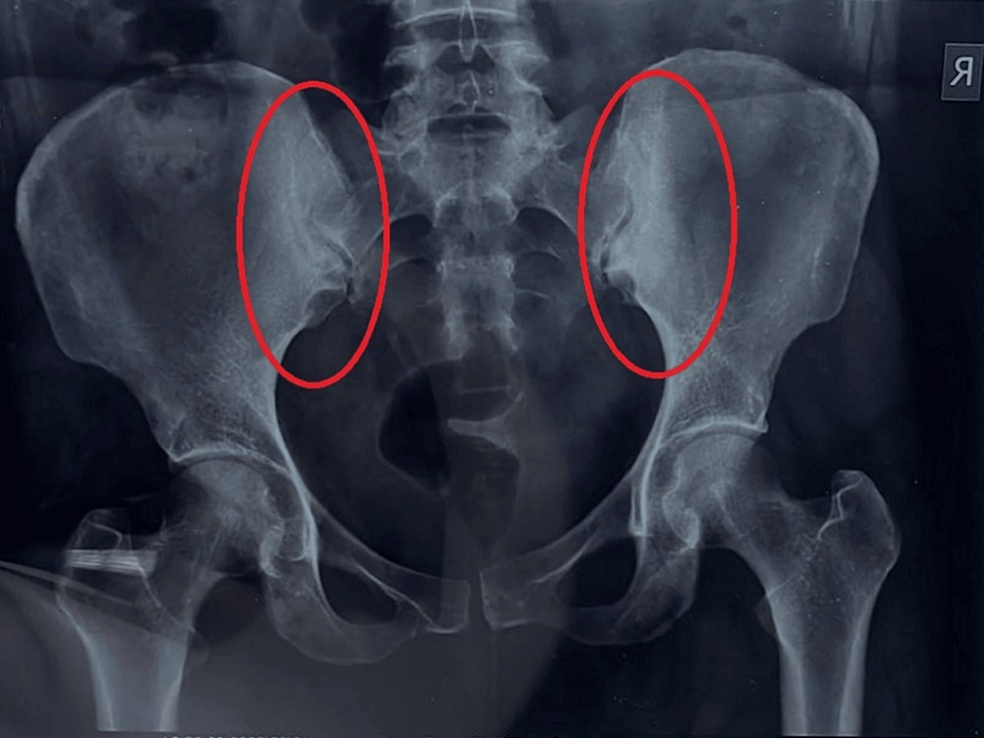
X-ray of the pelvis showing the sclerosis of the iliac part of sacroiliac joints without any erosion, irregularity, or fusion of sacroiliac joints
